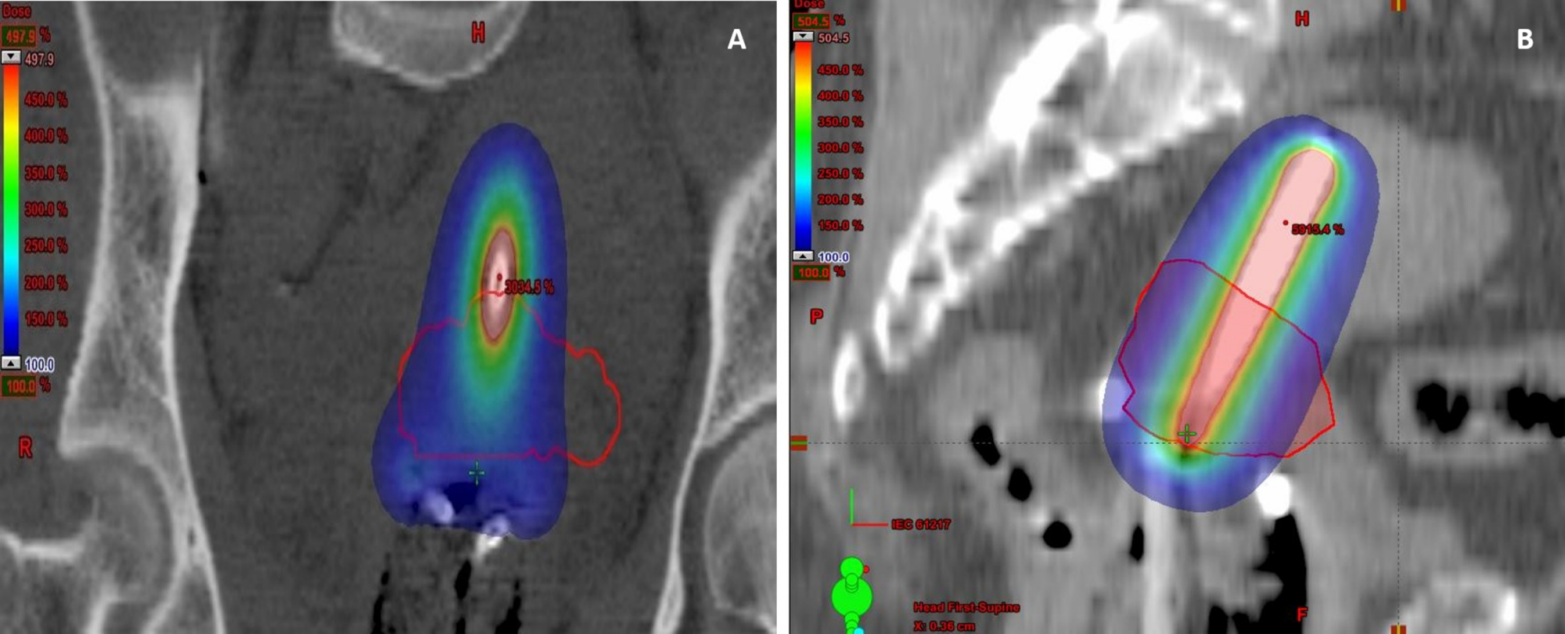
CT image (A, B) showing inadequate coverage of HRCTV (>25cc) by 100% isodose color wash HRCTV-High Risk Clinical Target Volume
